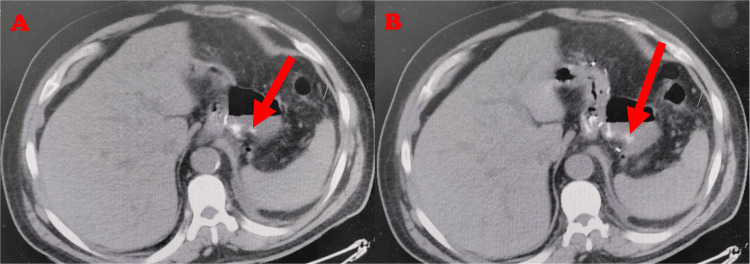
CT contrast extravasation Follow-up CT abdomen with oral and IV contrast (Day 5). (A) Axial CT demonstrating a large peri-gastric collection at the operative bed with an internal air-fluid level. The red arrow points to the air-fluid level within the collection at the staple-line site. (B) Consecutive axial slice demonstrating active oral contrast extravasation from the superior aspect of the residual stomach into the peri-gastric space. The red arrow highlights the point of active contrast leakage, confirming a communicating staple-line leak.
